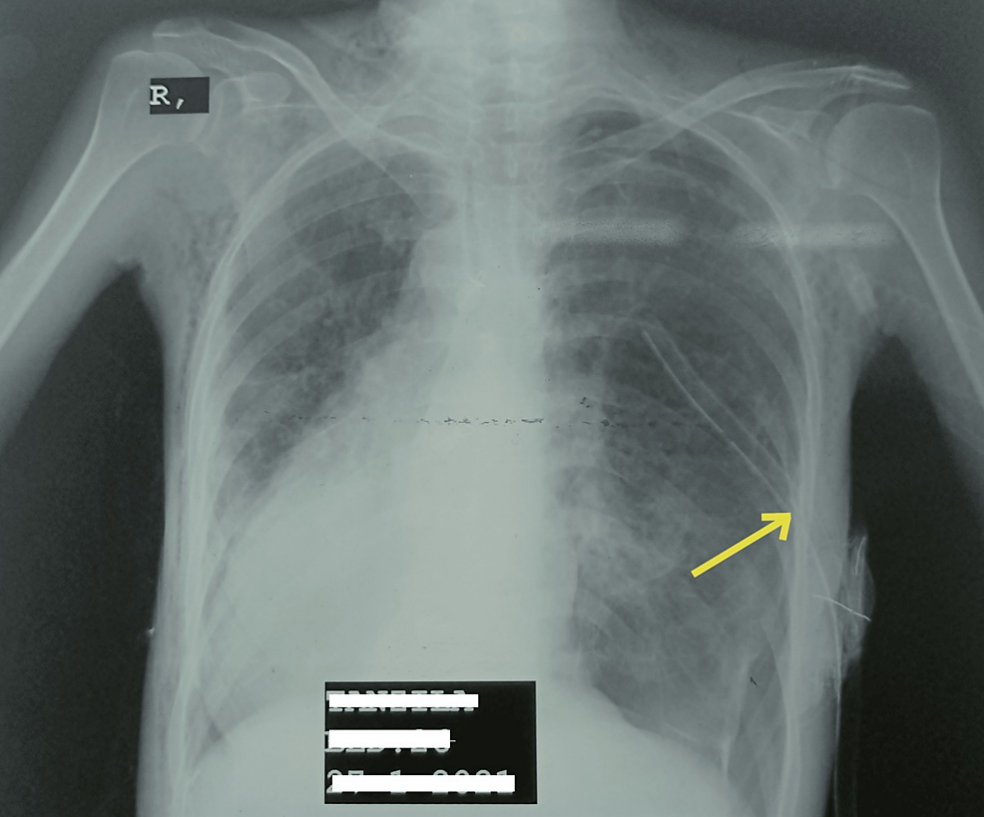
Chest radiograph revealing successful chest tube insertion
